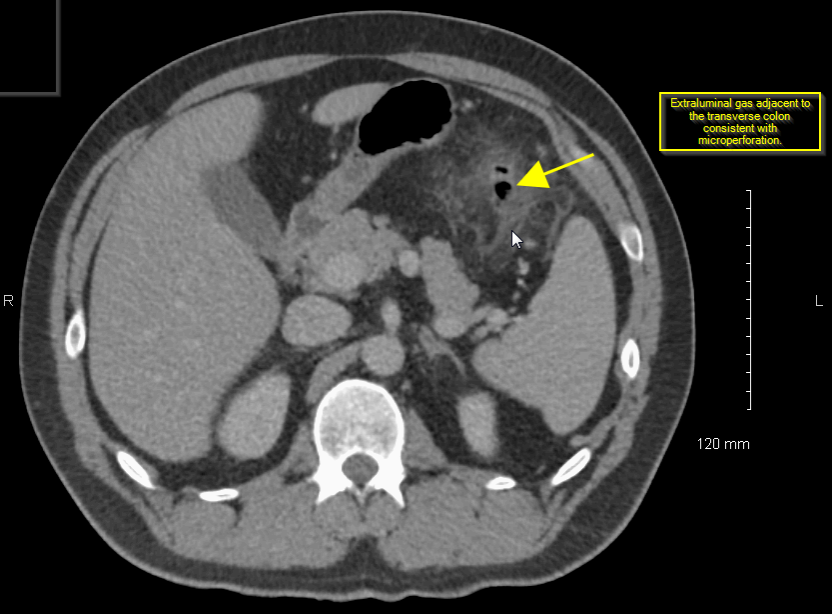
CT with Contrast of Patient Showing Transverse Diverticular Disease with Micro-Perforation (Yellow Arrows) Obtained in ED
